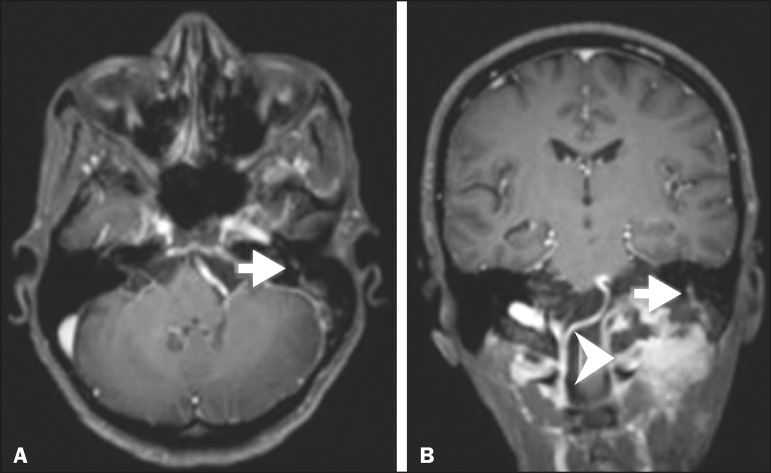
T1-weighted MRI scans of a 57-year-old female with (postoperative)
